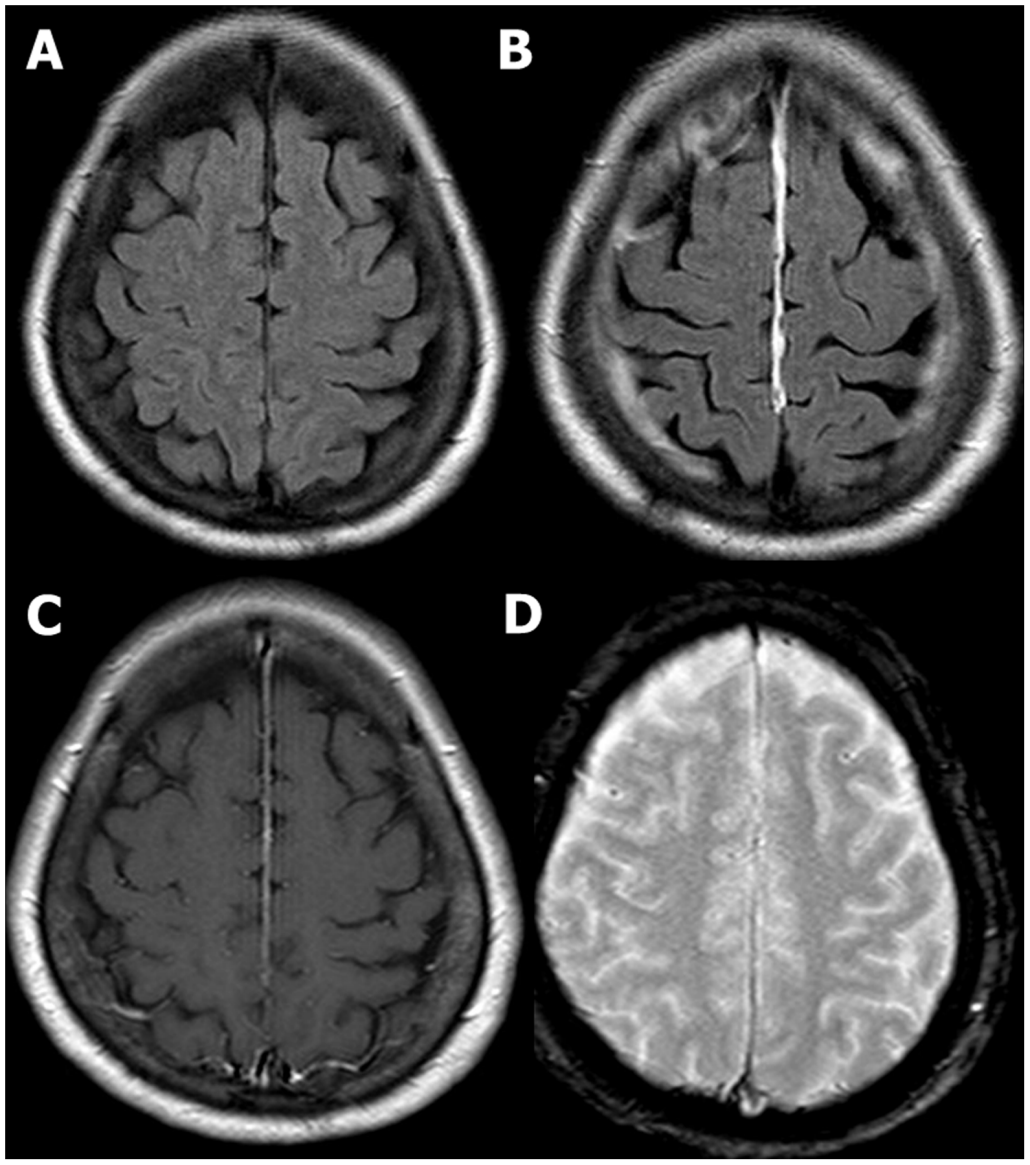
A 63-year- old female patient with a history of fall down 2 days ago. Initial GCS score was 15. She had transient episode of loss of consciousness less than 30(B) Only contrast-enhanced FLAIR MR image reveals abnormal finding – meningeal enhancement along falx. No demonstrable abnormality was found on unenhanced FLAIR (A), contrast-enhanced T1 weighted (C) and GRE (D) MR images.
